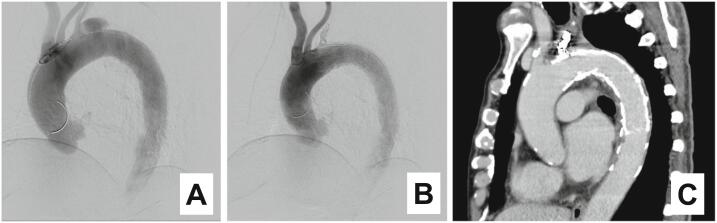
(A) Pre-TEVAR Aortography; (B) post-TEVAR Aortography; (C) post-TEVAR CT. TEVAR, thoracic endovascular aortic repair; CT, computed tomography.
